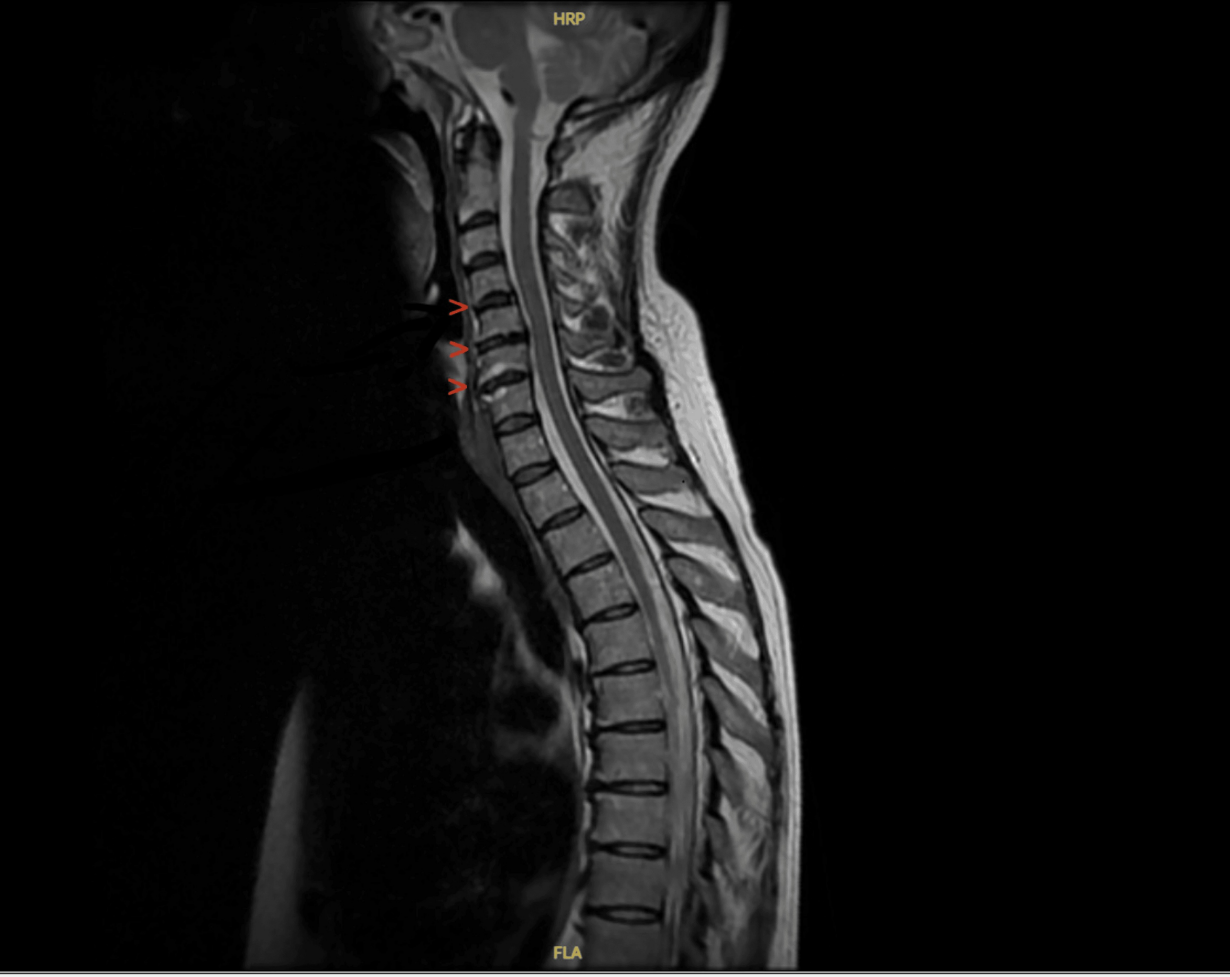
MRI whole spine showing degenerative changes between C5-C6 and C6-C7
